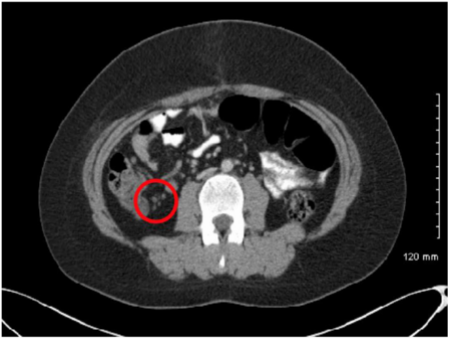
CT scan identifying the sub centimetre lymph nodes in right paracolic gutter.
